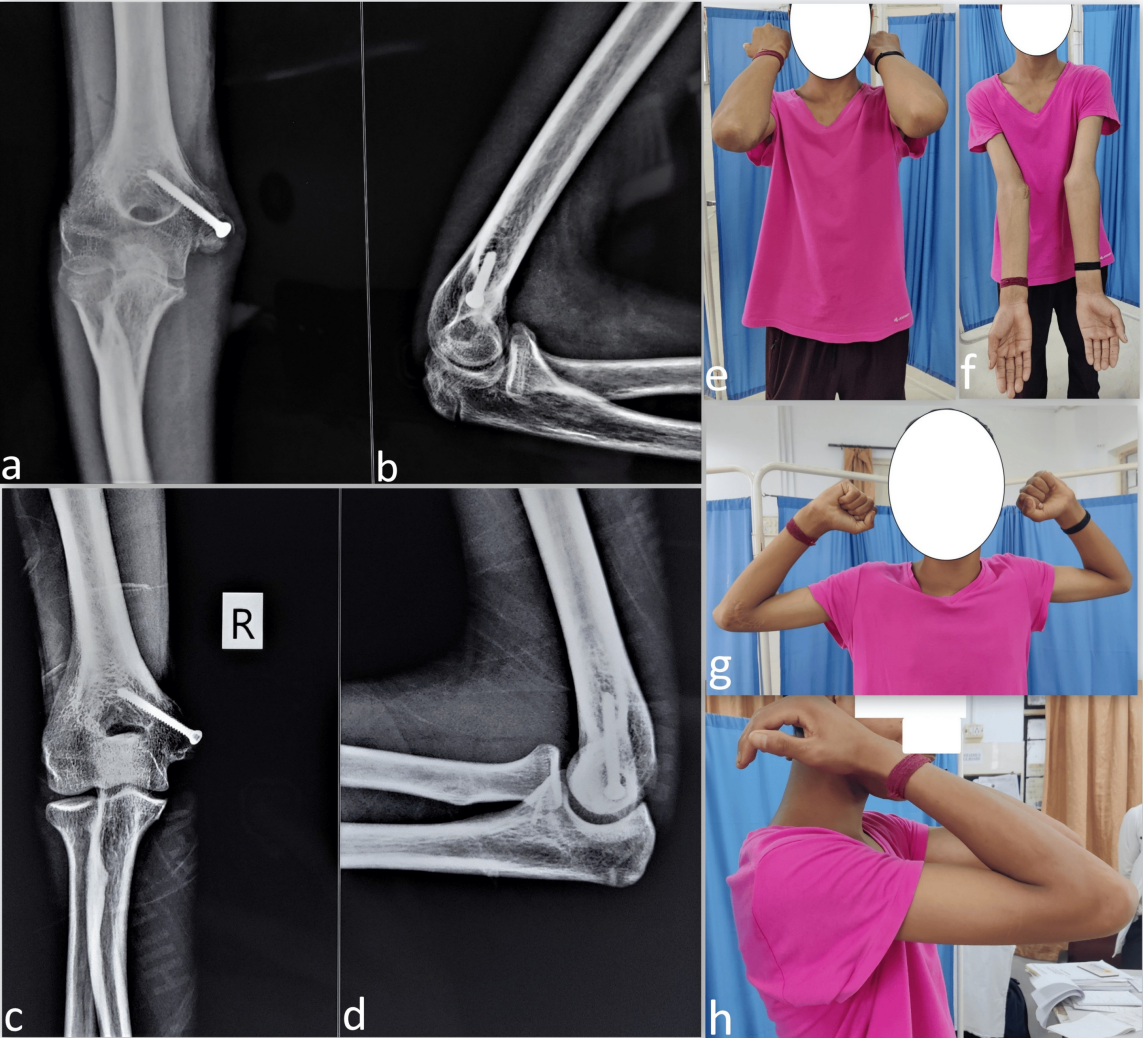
The radiograph at the 6th (a, b) and 13th-month (c, d) follow-up showing gradual union of the fracture along with good functional outcomes and regain of normal elbow movements with no elbow deformity (e, f) and full flexion-extension (g, h).
